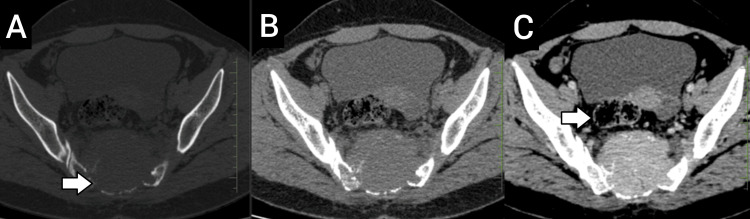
Lumbopelvic CT scan in axial view showing the lesion with destruction of the pelvic surface of the sacrum: (A) bone window, (B) non-contrast scan, and (C) contrast-enhanced scan, demonstrating slight anterior displacement of the rectum
